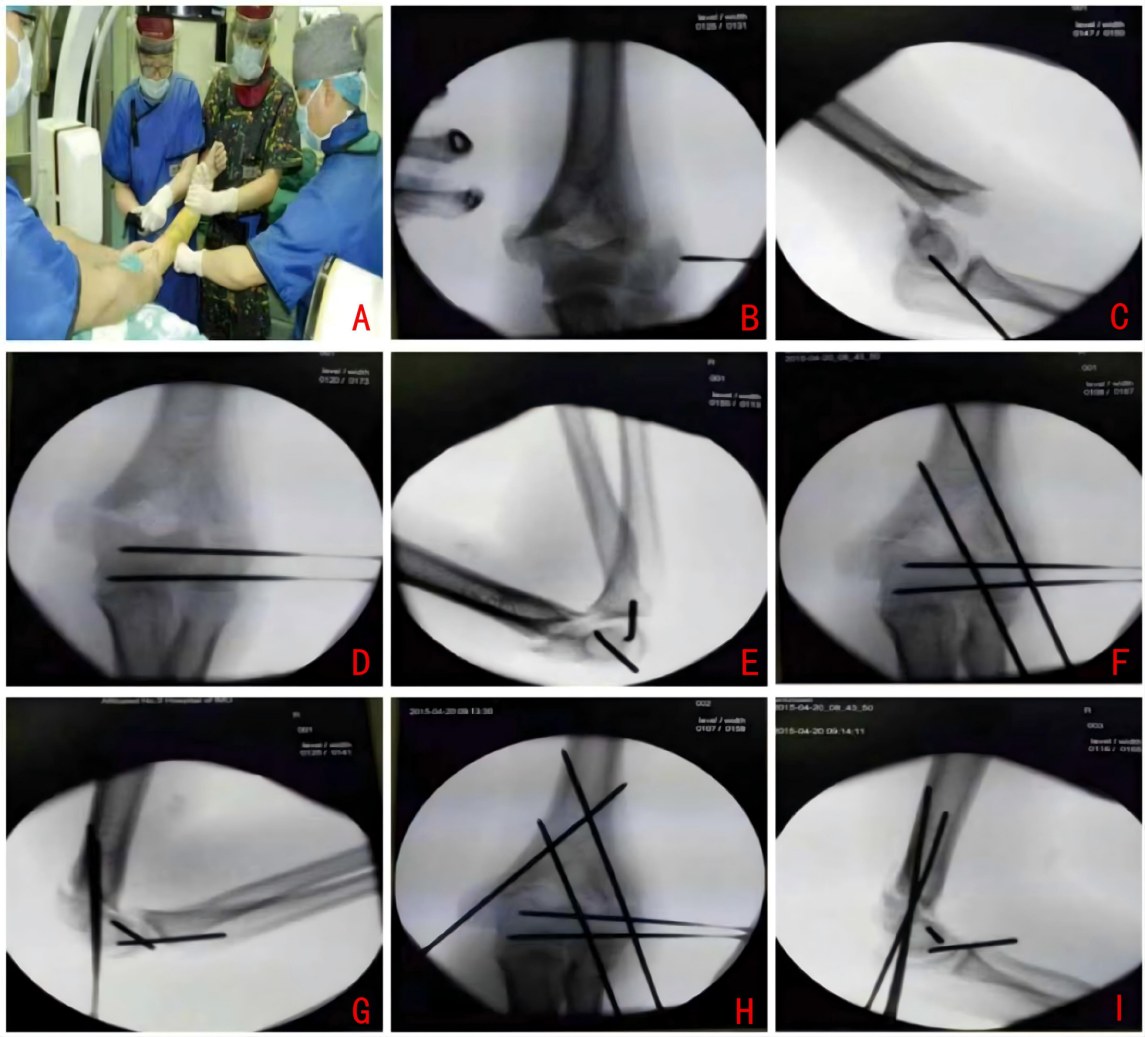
(A) Traction reduction. (B,C) Reduction via the “Leverage Principle” (Joystick Technique). (D,E) Conversion from intercondylar to supracondylar fracture pattern. (F,G) Supracondylar fixation. (H,I) Immediate postoperative radiographs.
